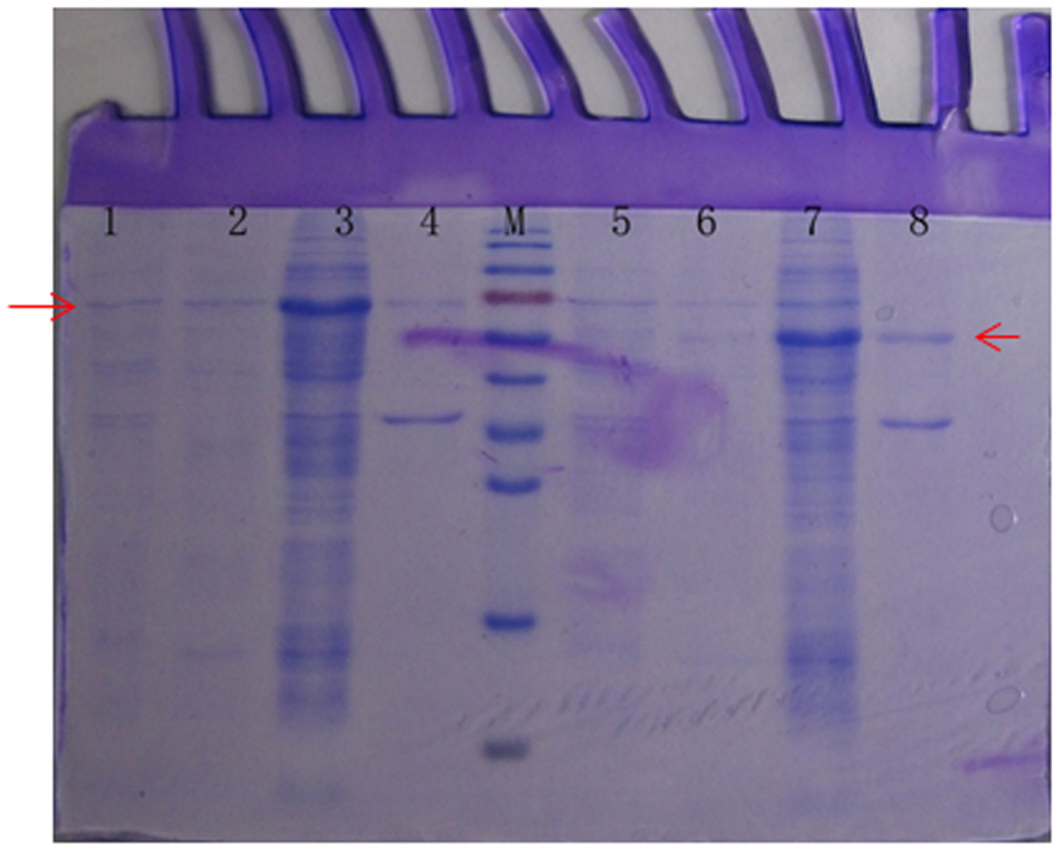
SDS-PAGE analysis of supernatant or inclusion bodies after ultrasonic disruption. Lane M: Protein Markers; lane 1: Supernatant after ultrasonic disruption of the tD4-producing cells that used binding buffer without the compounds; lane 2: Precipitation after ultrasonic disruption of the tD4-producing cells that used binding buffer without the compounds; lane 3: Supernatant after ultrasonic disruption of the tD4-producing cells that used binding buffer containing the compounds β-mercaptoethanol, urea, Tween 20, glycerol, and SDS; lane 4: Precipitation after ultrasonic disruption of the tD4-producing cells that used binding buffer with the compounds; lane 5: Supernatant after ultrasonic disruption of the tD5-producing cells that used binding buffer without the compounds; lane 6: Precipitation after ultrasonic disruption of the tD5-producing cells that used binding buffer without the compounds; lane 7: Supernatant after ultrasonic disruption of the tD5-producing cells that used binding buffer with the compounds; lane 8: Precipitation after ultrasonic disruption of the tD5-producing cells that used binding buffer with the compounds.
